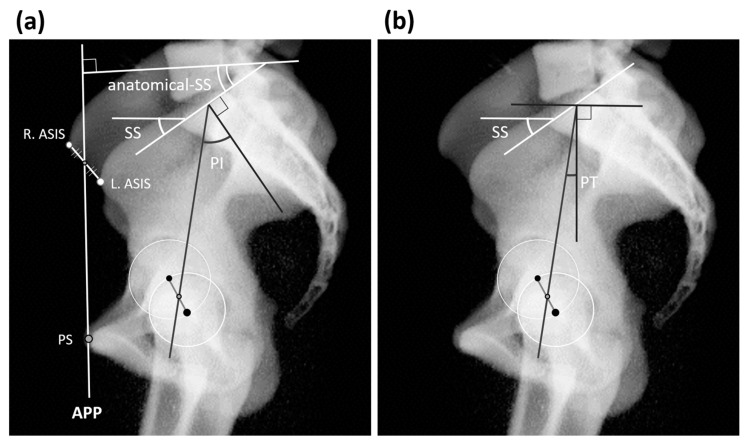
(a) Parameters of the pelvis. Radiograph was taken with the patient in a standing position. Sacral slope (SS) is the angle formed between the sacral plate line and the horizontal line perpendicular to the direction of the force of gravity. Anatomical SS (a-SS) is the angle formed between the S1 superior end plate and the line perpendicular to the anterior pelvic plane, which is defined as the line connecting the center point of the bilateral anterior superior iliac spine (ASIS) to the pubic symphysis. Left anterior iliac spine (L-ASIS). Right anterior superior iliac spine (R-ASIS). Anterior pelvic plane (APP). (b) Pelvic tilt (PT) is the angle formed between a line connecting the center point of the sacral plate to the femoral head axis line and a vertical line parallel to the direction of the force of gravity. Pelvic incidence (PI) is SS+PT.
